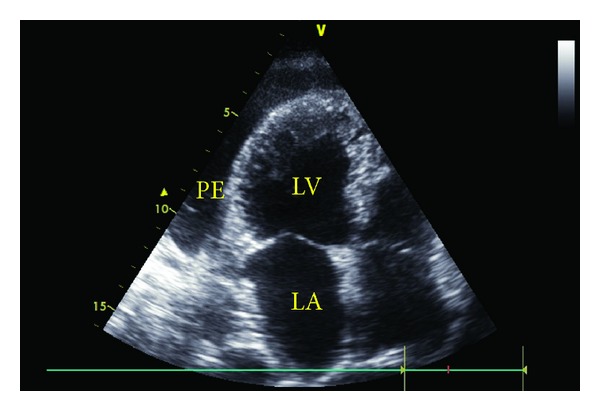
Apical four-chamber view with transthoracic echocardiography (PE: pericardial effusion, LV: left ventricle, and LA: left atrium).
